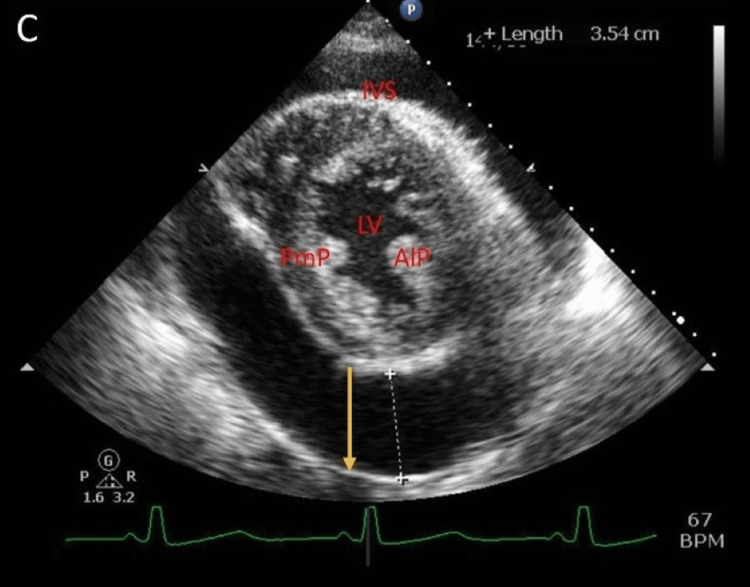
Transthoracic echocardiogram parasternal short-axis view with large pericardial effusion (yellow arrow). PmP: posteromedial papillary muscle; AIP: anterolateral papillary muscle; IVS: interventricular septum; LV: left ventricle.
